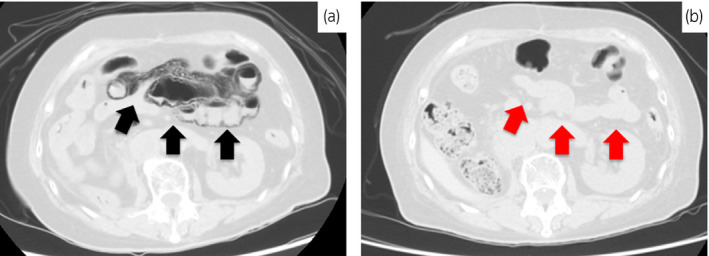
CT performed at the time of PCI diagnosis and on follow‐up. (a) CT performed to detect cause of vomiting. PCI is seen in a wide area around the ileocecal region (black arrow). There is no free gas or ascites. (b) CT on 7th day after discontinuation of sunitinib. PCI is reduced (red arrow).
